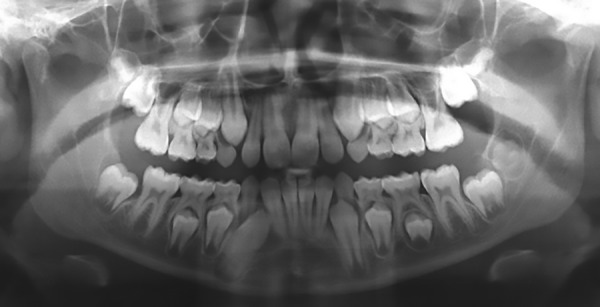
Panoramic radiograph image of the 18-month follow-up
